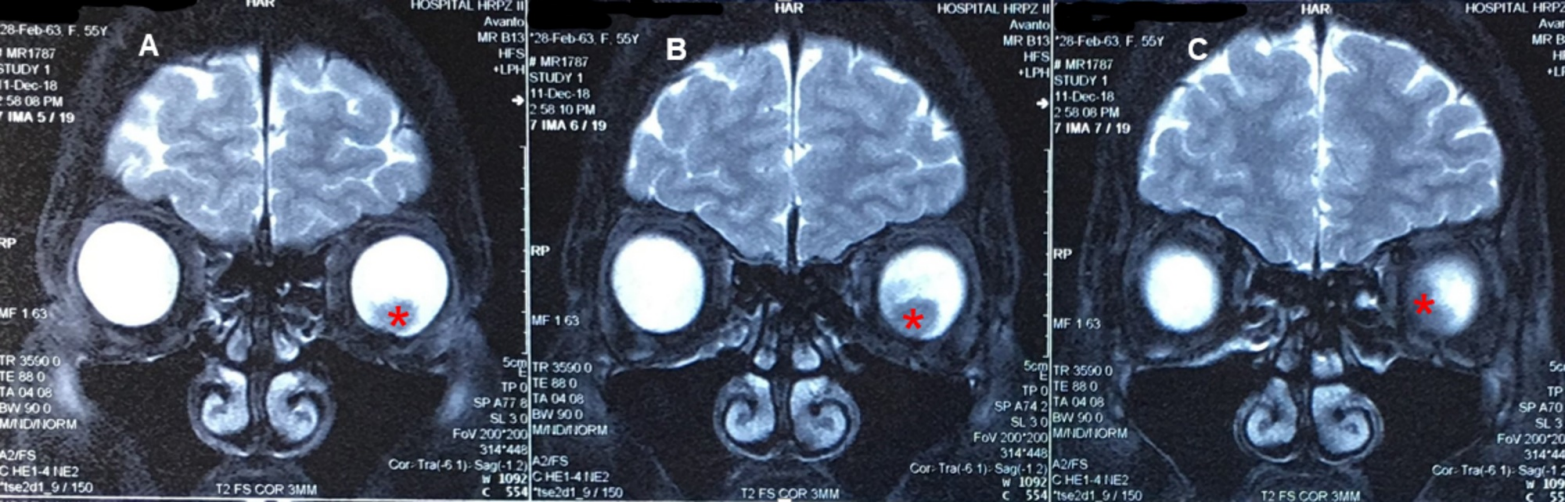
Coronal cut of MRI images taken at 3 mm intervals from anterior to posterior Image A (most anterior) showed the presence of a left intraocular mass inferiorly, appearing hypointense to vitreous in T2-weighted image (marked as *). In Image B, the lesion appeared larger in diameter and extended toward superonasal in a more posterior plane. Image C captured the mass at its posterior border. No extrascleral extension was noted in all planes.
